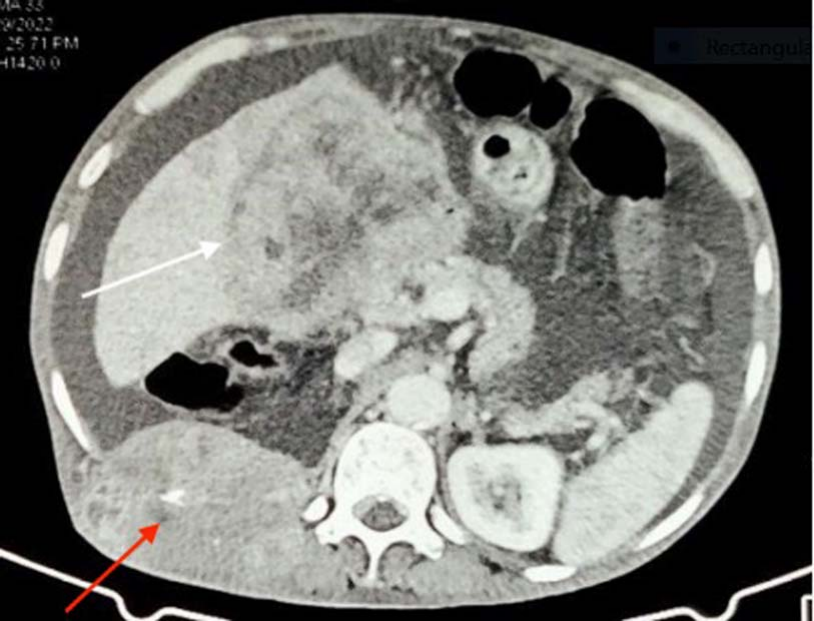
Axial contrast-enhanced abdomen CT shows well-defined heterogeneously enhancing liver mass (white arrow) and enhancing soft tissue density along with erosion of posterior rib (red arrow). CT, computed tomography.
